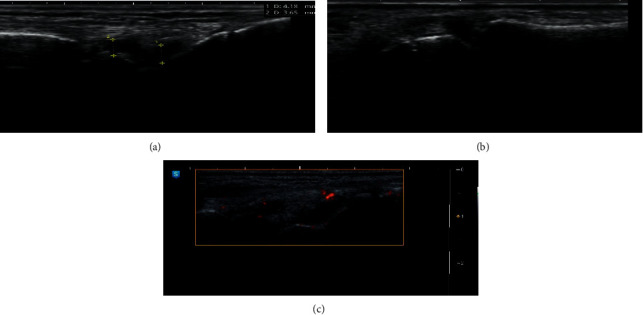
(a) Ultrasonographic examination of the wrist of an RA patient showing synovial thickness: at radiocarpal = 4.18 mm and intercarpal = 3.65 mm, (b) ultrasonographic examination of the wrist of an RA patient showing grade 2 carpal bone erosion, and (c) ultrasonographic examination of the wrist of an RA patient showing grade 2 Doppler activity.
